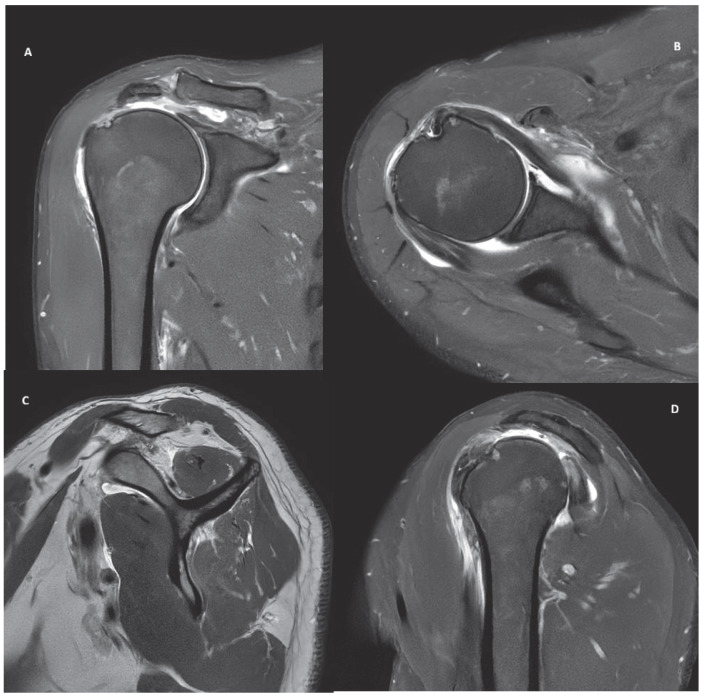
MRI images of a posterosuperior massive rotator cuff tear involving both supraspinatus and infraspinatus tendons in coronal (A), axial (B) and sagittal (C,D) view.
